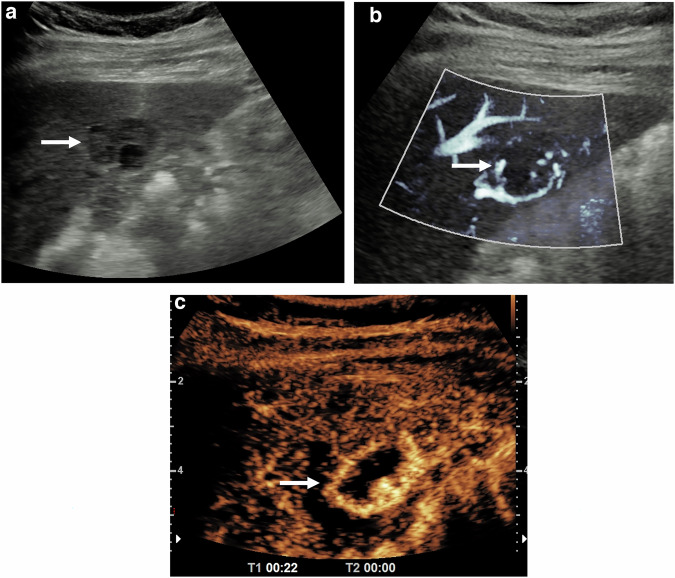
An atypical focal liver lesion on a background of hepatic steatosis, in a patient referred from a primary care physician with abdominal pain; cavernous haemangioma. a On the B-mode ultrasound image, a mixed reflective focal lesion (arrow) is noted, without definable characteristic features. b Microvascular imaging depicts some “puddling” of vascular flow (arrow), raising the possibility of an atypical haemangioma. c The contrast-enhanced ultrasound examination at 22 s, depicts the characteristic globular peripheral enhancement of a haemangioma
